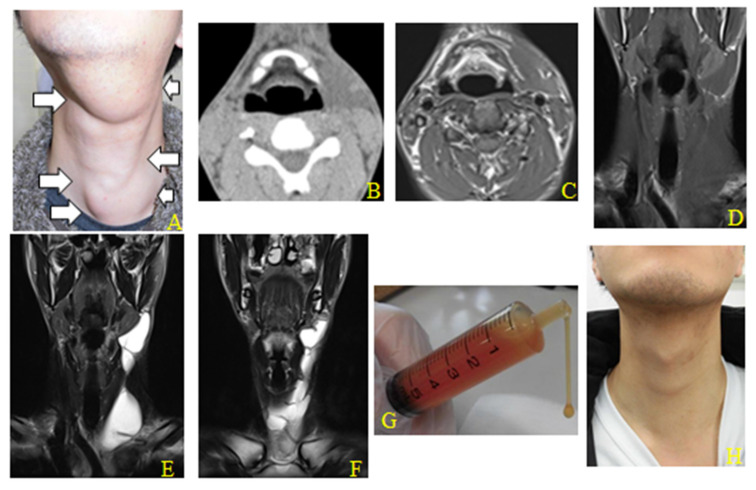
Totally shriveled right cervical lesion in a 22-year-old Japanese man after single treatment with OK-432. (A) Local findings before OK-432 treatment, showing the ranula intruding into the left anterior cervical region (16.4 cm) (arrow indicating the diffuse swelling of the anterior cervical region). (B)Initial axial computed tomography before OK-432 treatment showing the ranula intruding into the anterior cervical region. (C,D) Initial axial and coronal T1-weighed magnetic resonance image before OK-432 treatment, showing the ranula intruding into the anterior cervical region. (E,F) Initial coronal T2-weighted magnetic resonance image before OK-432 treatment, showing the ranula diving into the submandibular region. (G) The lesion puncture fluid was very sticky mucus. (H) Local findings 6 weeks after OK-432 treatment showing totally shriveled lesion.
